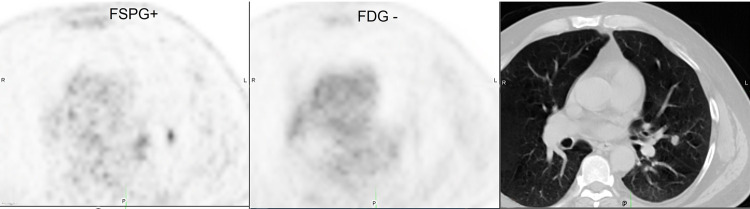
Subject with positive ^18^F-FSPG (SUVmax 2.02) and negative ^18^F-FDG (SUVmax 0.7) PET/CT.
